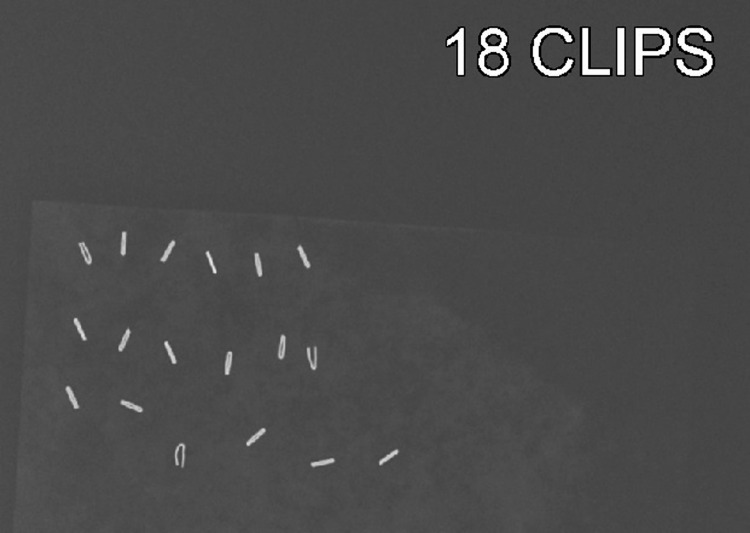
X-ray of 18 surgical clips removed during the procedure.
